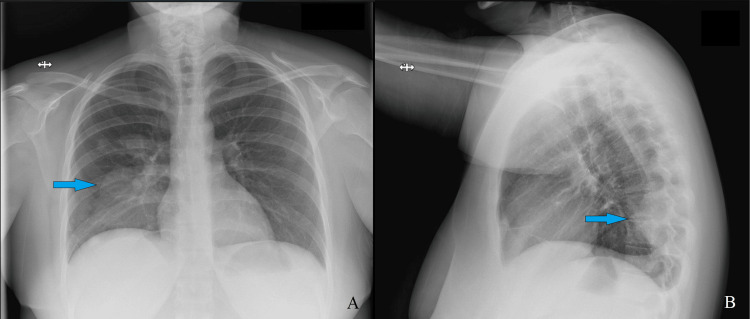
Chest X-ray on admission Posterior-anterior (A) and lateral (B) chest X-ray with arrows showing the right lower lobe opacity.
